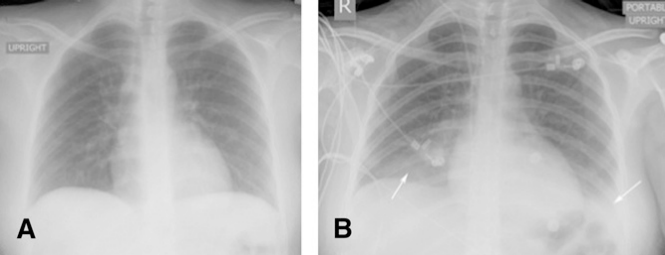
(A) Chest radiograph at initial presentation and (B) after 24 hours showing bilateral pleural effusions (white arrows).
